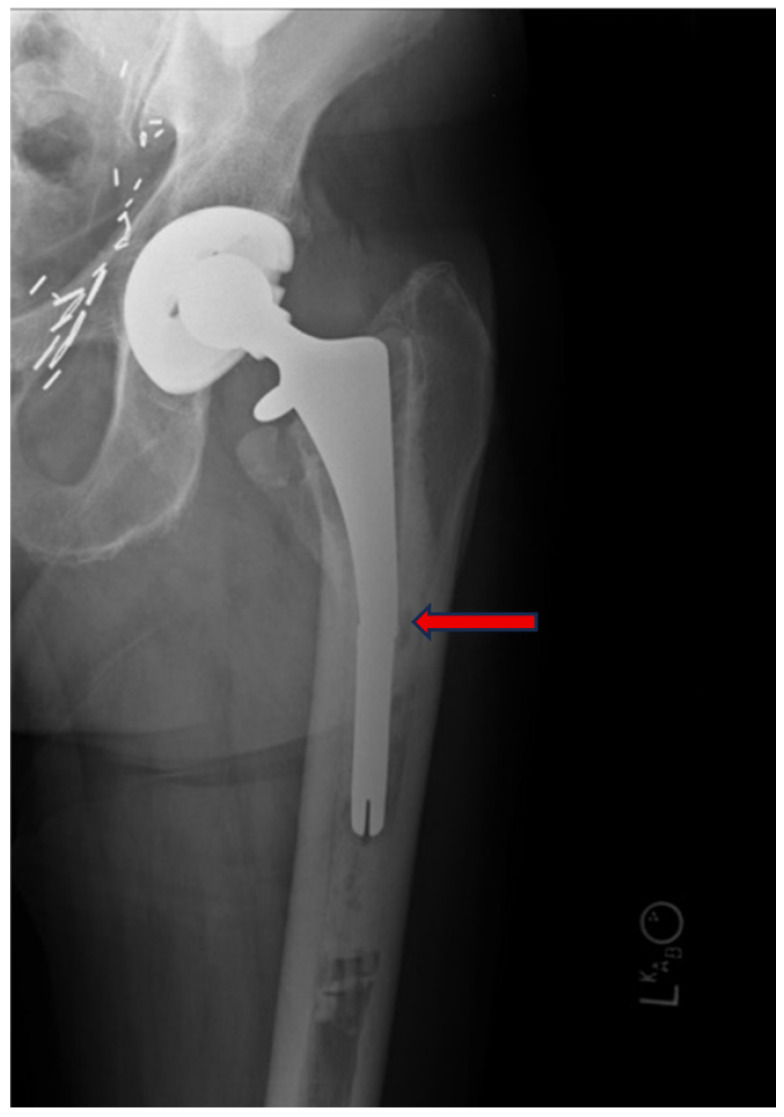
X-ray of the hip implant fracture location, denoted by the red arrow.
